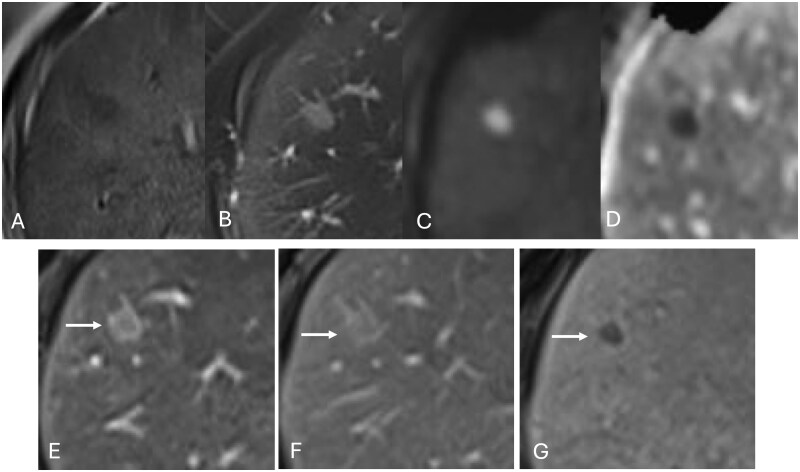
(A-C) A lesion in S8 showed hypointense on a T1-weighted image (A) and hyperintense on a fat-suppressed T2-weighted image (B) and diffusion-weighted imaging (C). (D) The ADC map of the tumor indicated an ADC value of 0.85 × 10^−3^ mm^2^/s. (E-G) On gadoxetic acid-enhanced (EOB) MRI, the lesion showed a faint enhancement in the arterial phase (E), appeared isointense relative to the surrounding liver parenchyma in the portal phase (F), and showed hypointense in the hepatobiliary phase (G). The peri-tumoral regions demonstrated early enhancement in the arterial phase (arrow in E), with persistent enhancement in the portal phase (arrow in F), and showed isointense to the surrounding liver parenchyma in the hepatobiliary phase (arrow in G).
